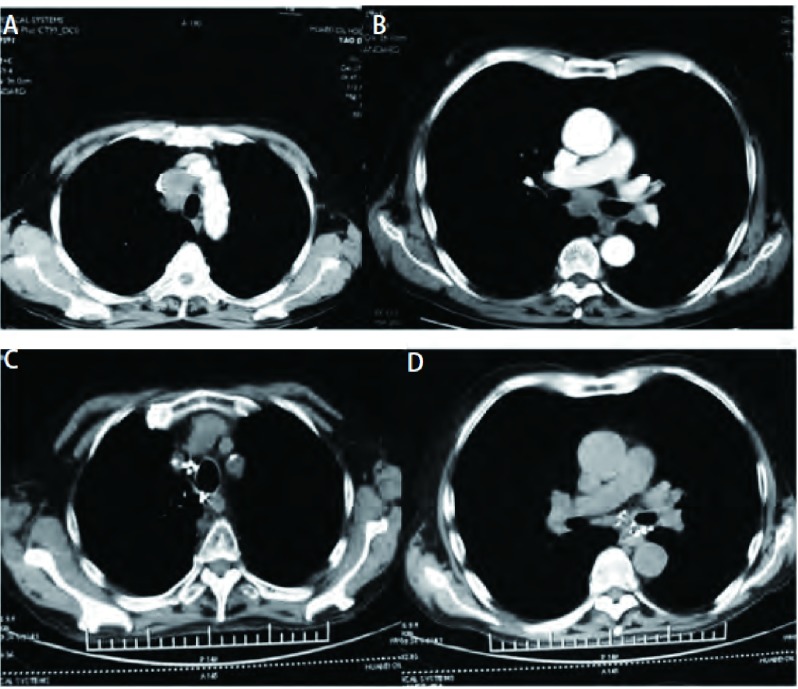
纵隔淋巴结转移癌。A, B:治疗前CT增强扫描显示4R、7区淋巴结转移癌; C, D:治疗后6个月复查病灶PR。 Lymph node metastases in mediastinum.A, B:Enhanced CT scanning shows the lymph node metastases of 4R and 7 regions before therapy; C, D:6 months after radioactive seeds implantation, tumor outcome is PR.PR:Partial response.
